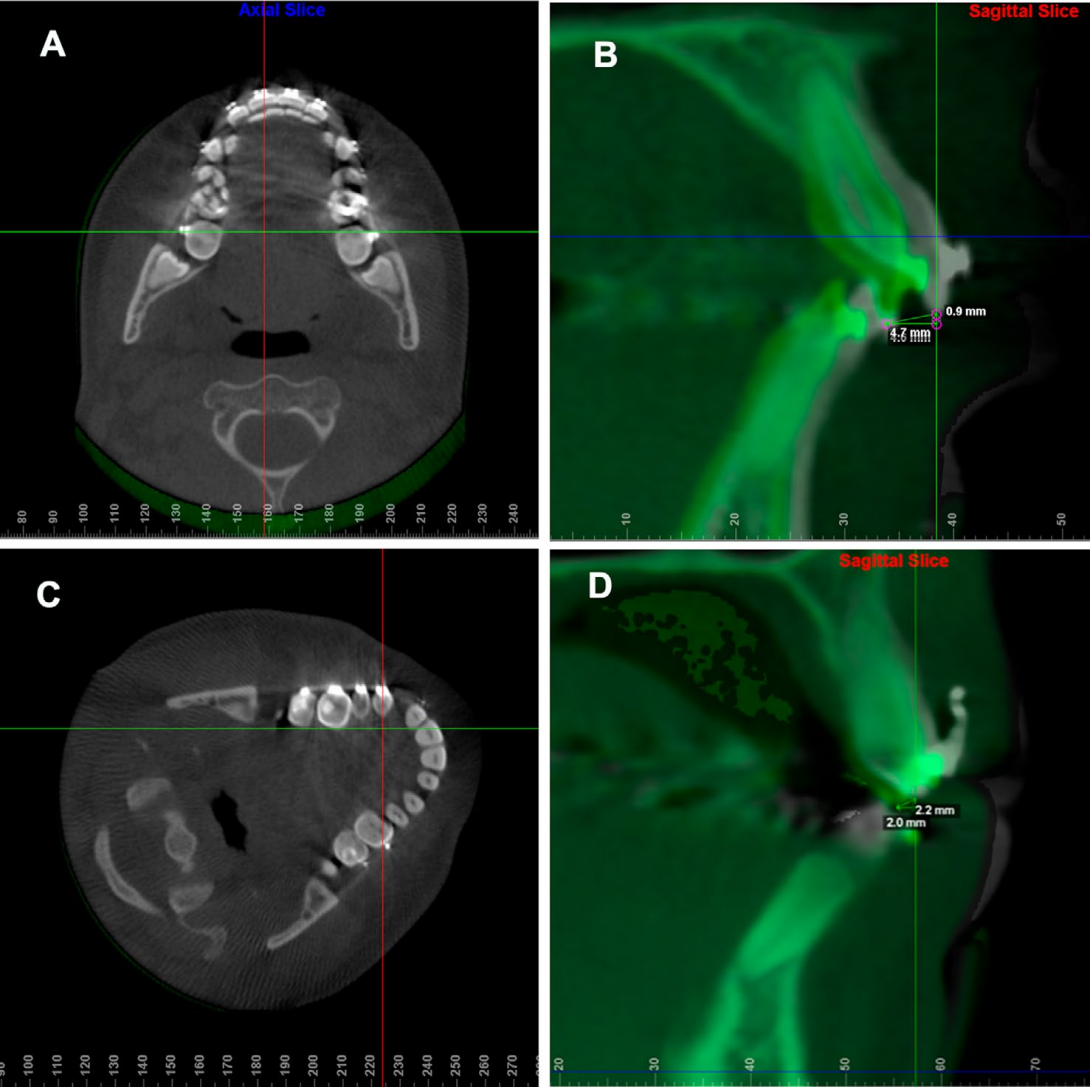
CBCT measurements using Dolphin Imaging software. (A) in the axial view, selection of the cut that passes through the center of the incisal edge of the maxillary right central incisor (red line) at T0; (B) in the sagittal view, measurement of 2D and horizontal displacement from the lowest point of the incisal border from T0 (grey) to T1 (green); (C) in axial view, after rotating the initial CBCT, selection of the slice that passes through the center of the crown of the maxillary right cuspid (red line); (D) in the sagittal view, measurement of 2D (combination of horizontal and vertical displacement) and the pure horizontal displacement of the lowest point of the incisal border from T0 (grey) to T1 (green).
